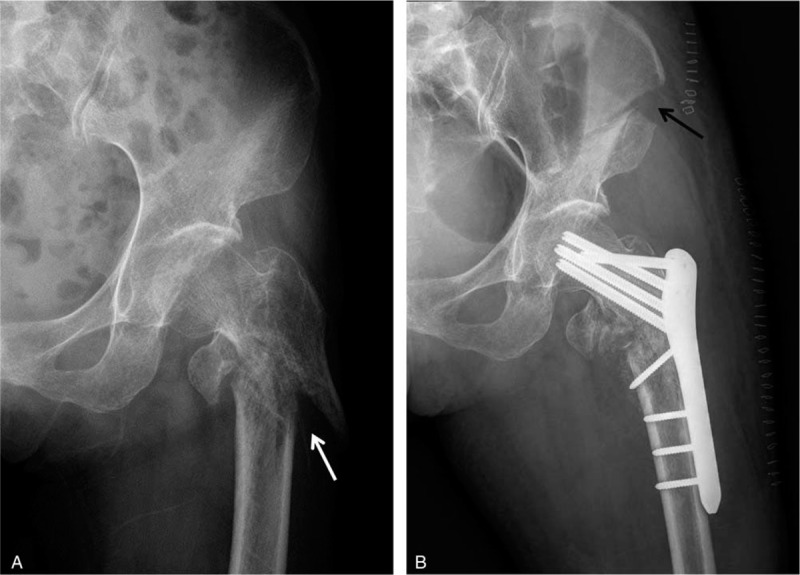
Plain radiography. (A) Delayed AO/OTA 31-A3 left femoral intertrochanteric fracture (white arrow). (B) Proximal femoral locking plate supplemented with anterior iliac crest bone graft harvested (black arrow).
